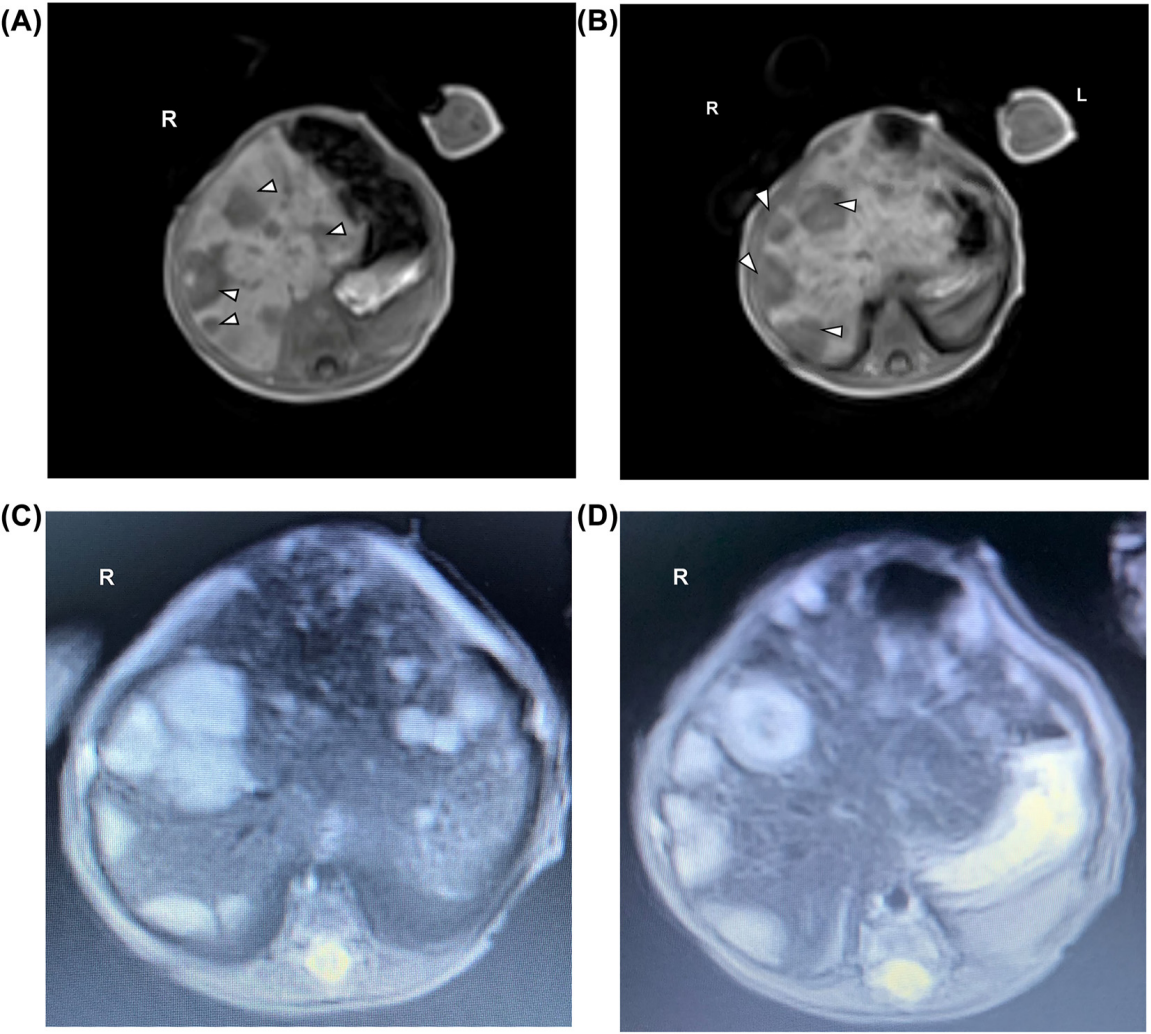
Coronal T1-weighted magnetic resonance imaging (MRI). (A, B) Coronal T1-weighted magnetic resonance imaging (MRI) scan post intravenous contrast agent shows diffusely, heterogeneous enhancement through the liver with distinctly nodular foci of hypointensity (arrows). (C, D) Coronal T2-weighted magnetic resonance imaging (MRI) scan shows diffusely, heterogeneous appearing liver with distinctly nodular foci of hyperintensity (arrows).
